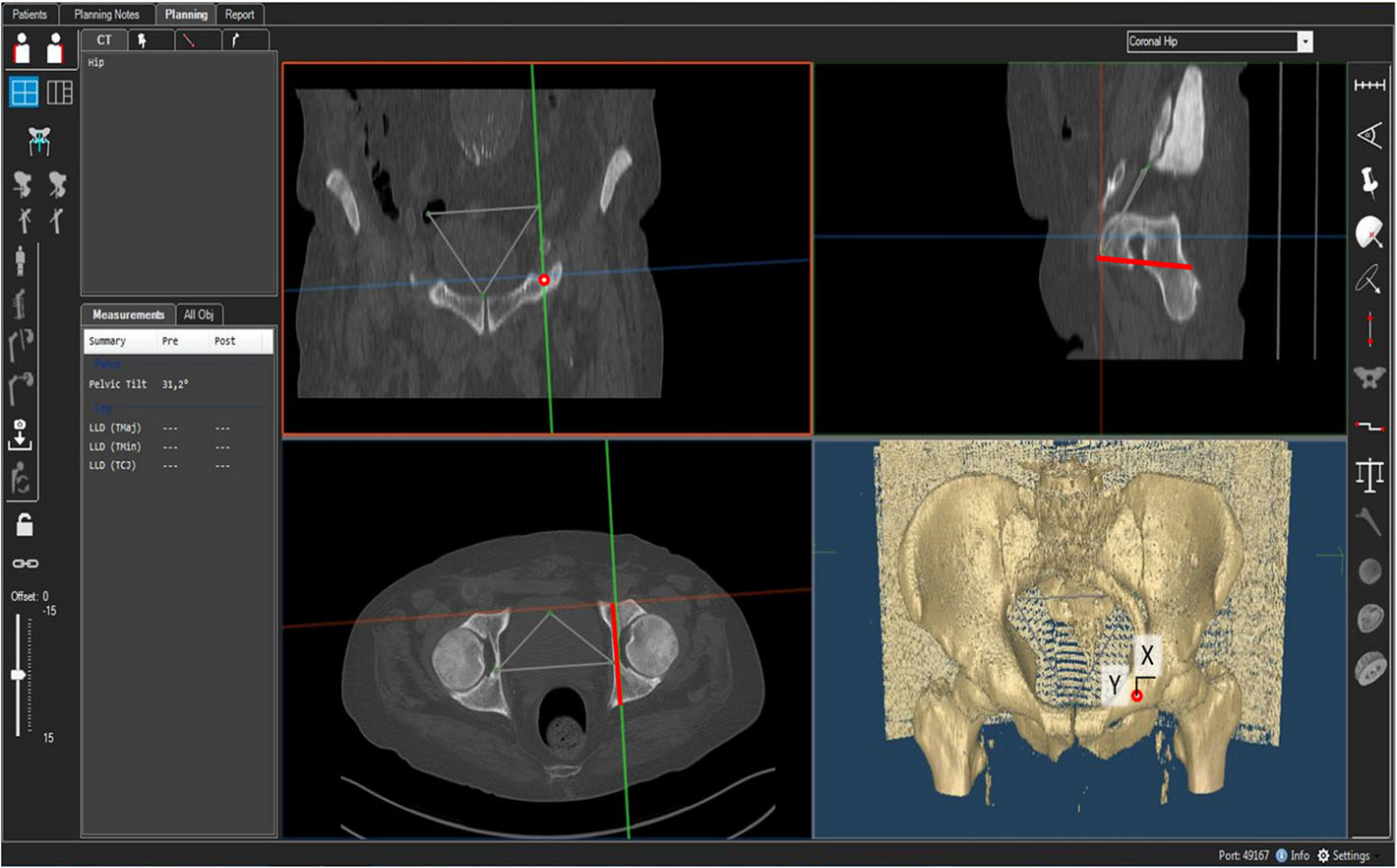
Setting of the 3D-CT-based measurement of the infra-acetabular screw position
